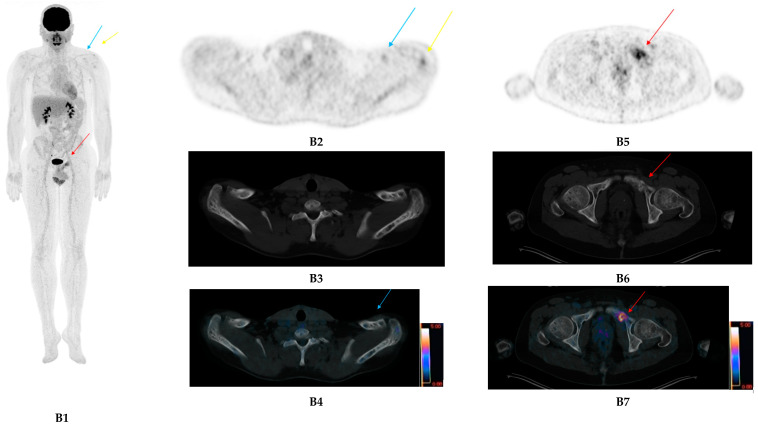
IgGk multiple myeloma (MM) patient at first relapse after autologous stem cell transplantation. Positron emission tomography with computed tomography using 18F-deoxyglucose (FDG-PET/CT) images before therapy (A) show several focal bone lesions (FLs) in maximum intensity projection (MIP) images (A1), especially the left pelvic FL (Red arrow; (A2) PET images; (A3): computed tomography (CT) images; (A4) fused images, maximum standardized uptake value (SUV_max_: 9)) and left collarbone (Blue arrow)/acromion FLs (Yellow arrow) in axial slices (A5/A6). Note the numerous osteolytic bone lesions without corresponding increased FDG uptake in the CT images as this patient had been pretreated, and morphological abnormalities may persist a long time after. FDG-PET/CT images after therapy (B) shows overall decrease in FL uptake in MIP images (B1). Whilst the left collarbone(Blue arrow)/acromion FL (Yellow arrow) residual uptake (B1/B2/B3/B4) is lower than the hepatic background (Deauville 3), the left pelvic FL (Red arrow) residual uptake (B1/B5/B6/B7) has decreased but is still higher than the hepatic background (Deauville 4, SUV_max_: 6) revealing a partial metabolic response.
